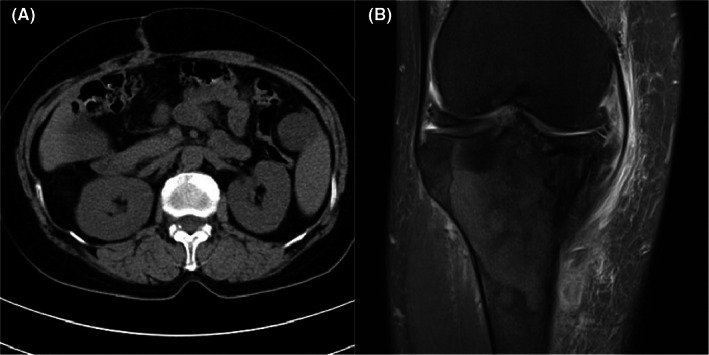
Recurrences in patient 3: (A) CT scan showed a recurrent tumor in the perisplenic region 14 months after surgery. (B) MRI showed a second recurrence in right tibial metaphysis and upside of right tibial shaft 26 months after surgery.
